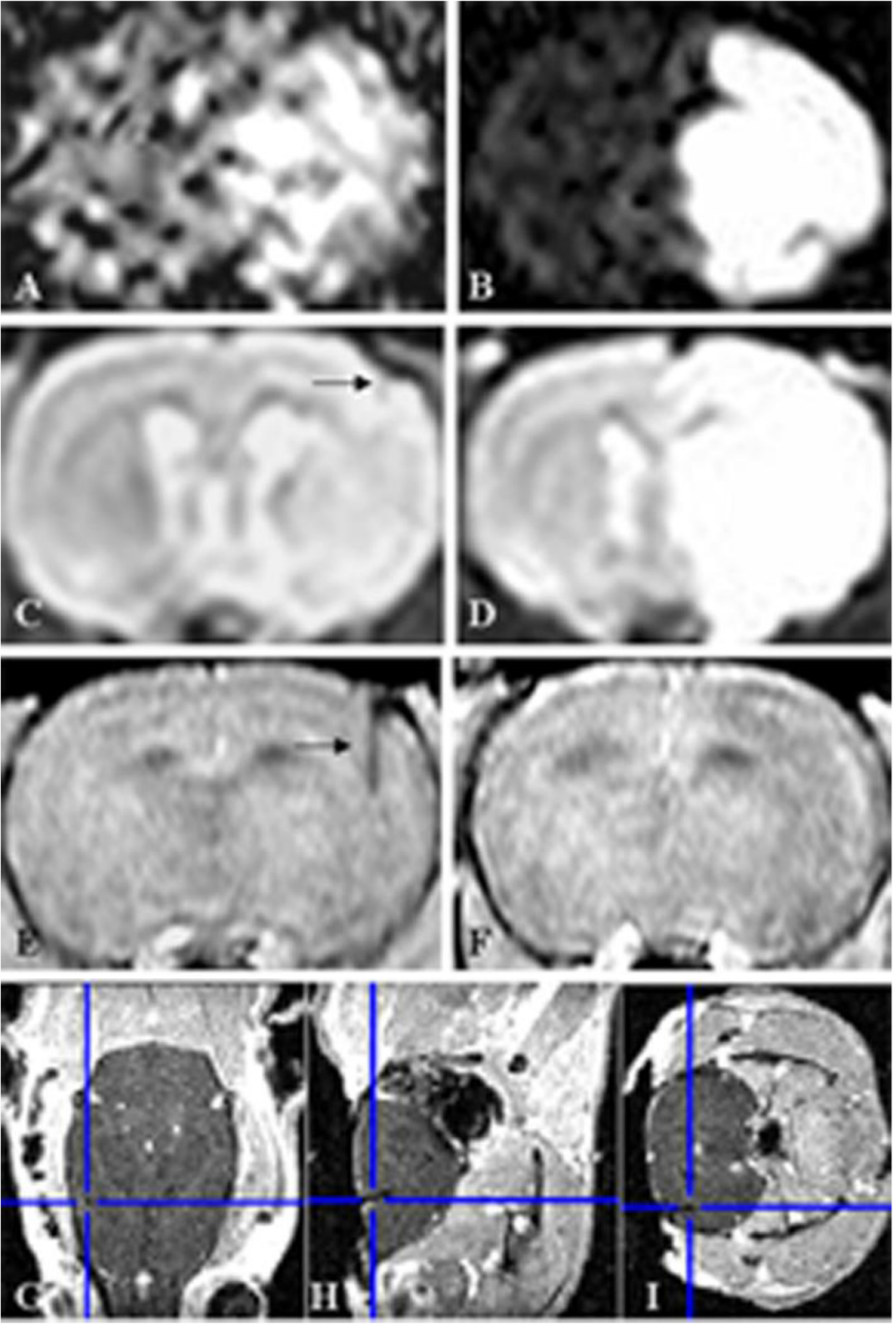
Magnetic resonance imaging showing localization of the pO_2 _detecting probe (Licox® CMP) and infarct lesion. A, C, E, DWI, T2WI, and time-of-flight (TOF) image after 90-min ischemia and 1-h reperfusion. B, D, F, DWI, T2WI, and TOF image after 90-min ischemia and 24-h reperfusion. G, H, I, three dimensional localization of the probe. DWI, diffusion-weighted images; T2WI, T2-weighted images.
